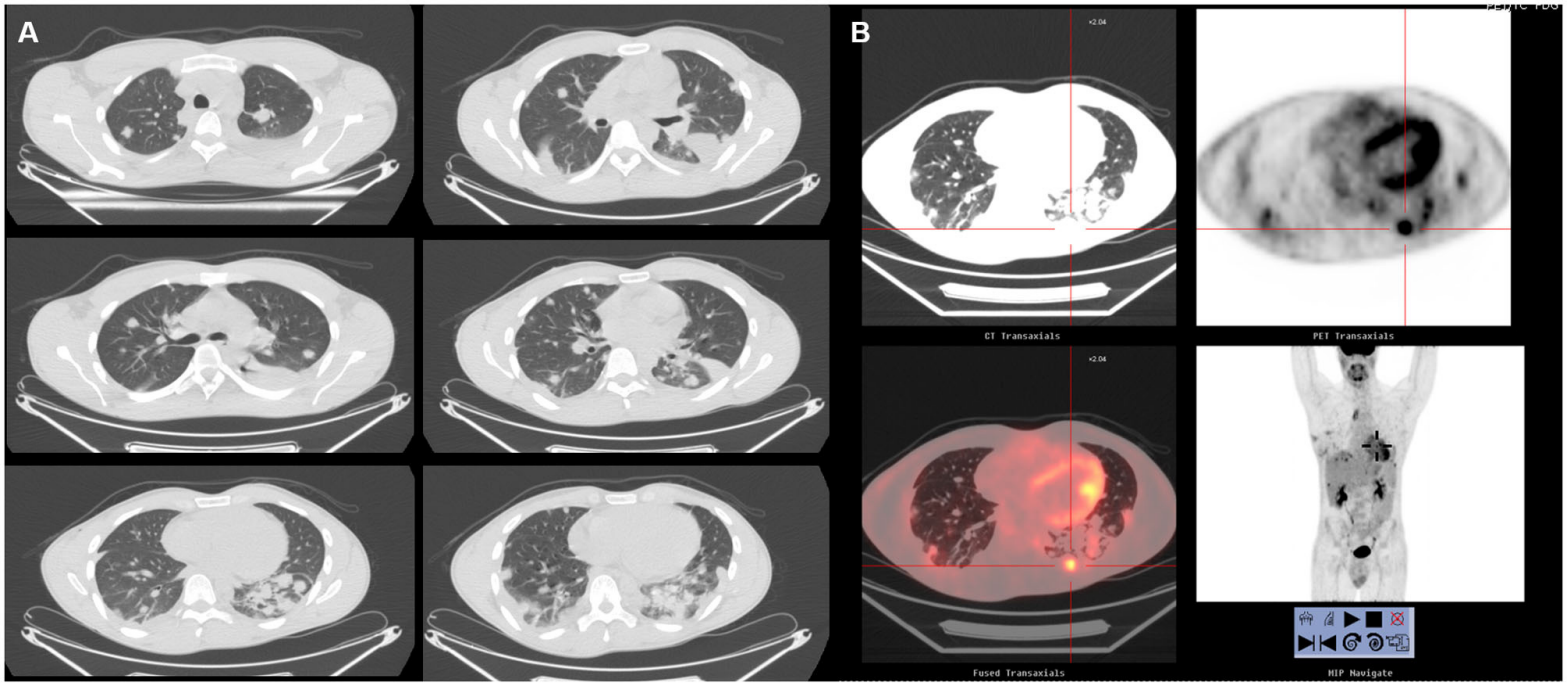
(A) Chest CT in case 1 before treatment. The lungs showed bilateral nodules. (B) Total body ^18^F-FDG PET. No hypermetabolic lesions other than the lesions in the lungs.
